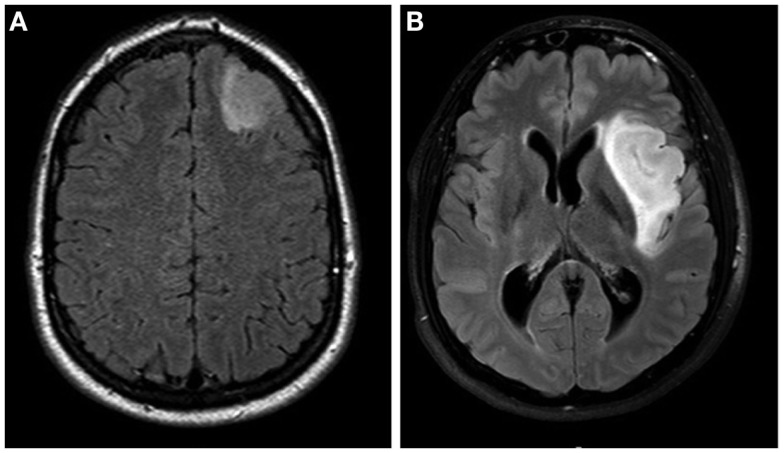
(A) MRI FLAIR sequence showing a left frontal astrocytoma grade II located in a non-eloquent area with mainly cortical location, in a 38-year-old female. (B) MRI FLAIR sequence showing an insular oligodendroglioma grade II, located affecting cortical and subcortical regions, in a 27-year-old male.
